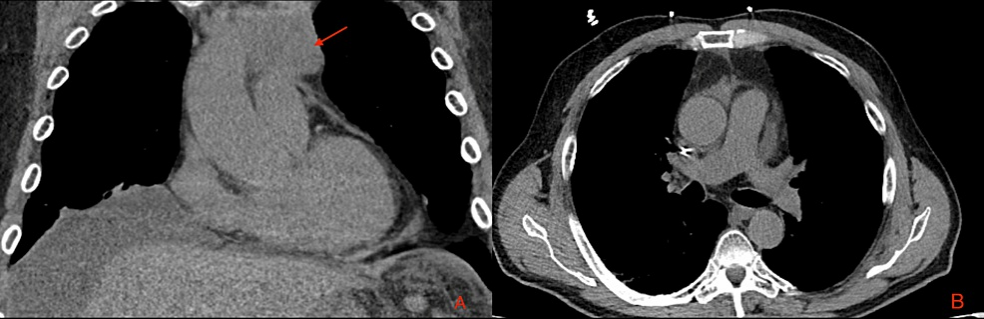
CT without contrast two weeks following chemoradiotherapy The images show an interval decrease in anterior mediastinal mass size (A) and resolution of main pulmonary artery compression (B) CT: computed tomography
